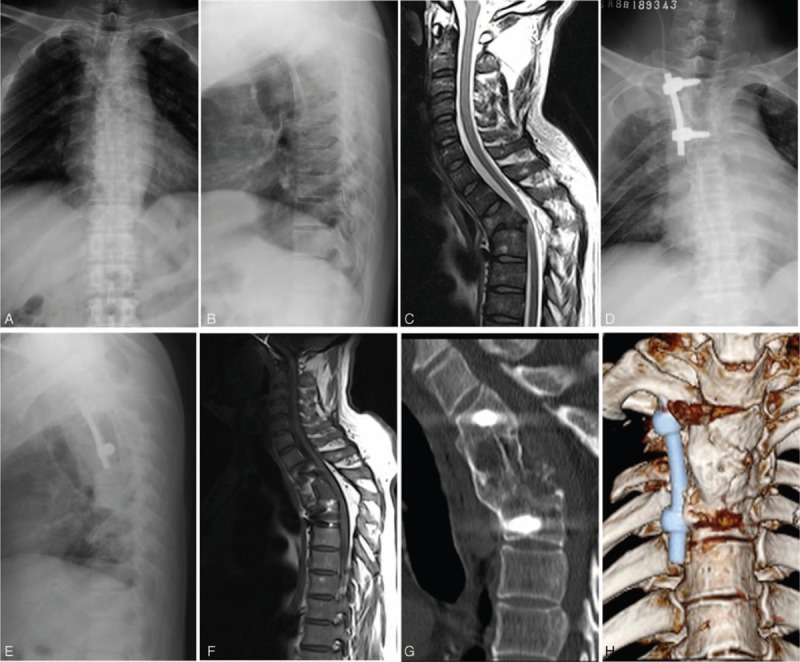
A 45-year-old man with spinal tuberculosis at T2 was treated by the subscapularis transthoracic approach. Preoperative anteroposterior and lateral X-ray imaging and sagittal MRI (A–C) showed T2 vertebral body destruction, collapse, disappearance of the intervertebral space, cord compression, and a local kyphosis angle of 44°. Postoperative thoracic lateral X-ray (D, E) of the same patient revealed good fixation location and a kyphosis angle of 37°. The 12-month follow-up examination showed satisfactory spinal decompression and no significant loss of kyphosis angle (36°) (F). The CT image and 3D reconstruction (G, H) showed that the bone graft had healed, with no lesion recurrence at T2.
